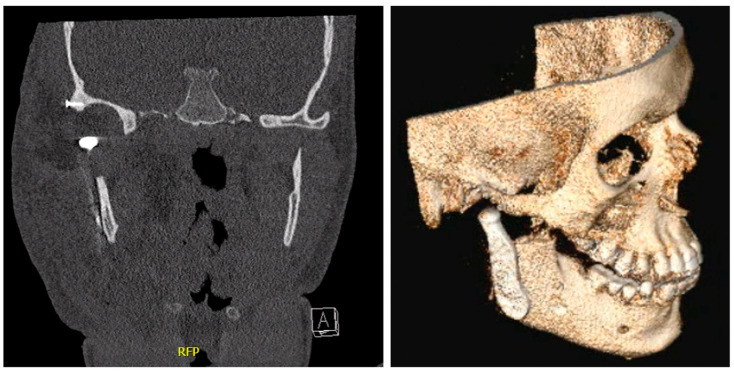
A CT scan and 3D animation one day after right-sided TMJ reconstruction.
